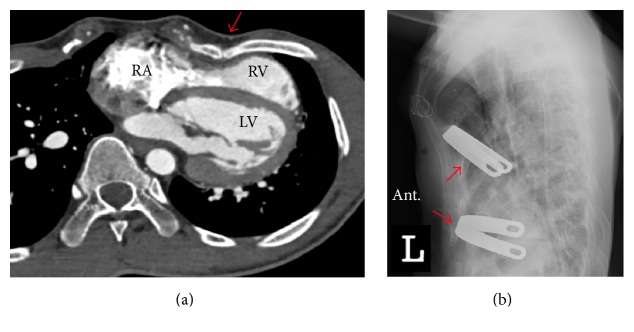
(a) Cross-sectional computed tomography image shows external compression (red arrow) on the basal-to-mid portion of the right ventricle (RV) and dilation of the right atrium and RV apical site (Haller index 5.21). (b) A lateral view of the chest X-ray after Nuss operation shows that anterior chest wall is decompressed by two Nuss bars underneath the sternum (red arrows) across the chest. RA, right atrium; RV, right ventricle; LV, left ventricle; ant., anterior.
